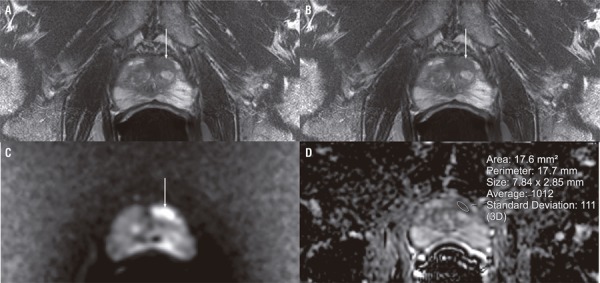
75-year-old man with suspected prostate cancer. Total serum PSA=8.5ng/mL. Prostate volume=41.2cc. Transition zone volume=24.3cc. PSAD=0.21. PSADtz=0.35. Images demonstrate a 1.2cm PI-RADS v2 4 lesion in the left apex anterior transition zone (arrows). Mean ADC value=1012x10−6mm2/s (region-of-interest on D). TRUS-MRI fusion biopsy diagnosed Gleason 3+4 prostate cancer. No other focus of high-grade prostate cancer was diagnosed on systematic biopsy. (A) T2-weighted image, (B) dynamic contrast enhanced (DCE) upslope parametric map, (C) diffusion-weighted image (DWI), (D) apparent diffusion coefficient (ADC) map.
